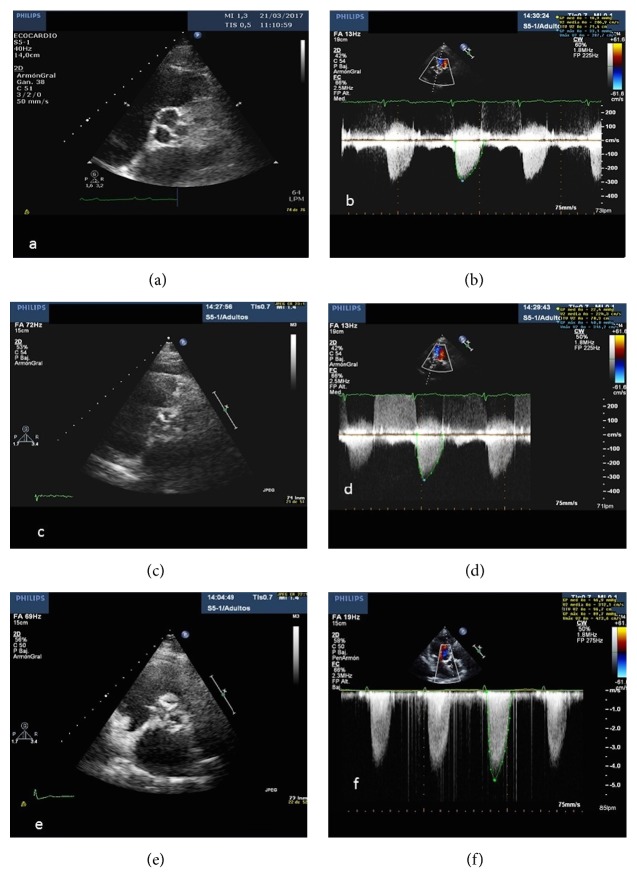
Two-dimensional echocardiogram, short axis view, and continuous Doppler showing mild (a and b), moderate (c and d), and severe (e and f) aortic valve stenosis, respectively.
